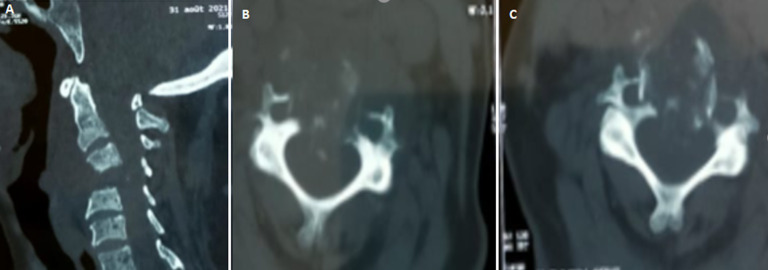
(A,B,C) cervical spine CT, lytic process centered on C4 with posterior wall recession
